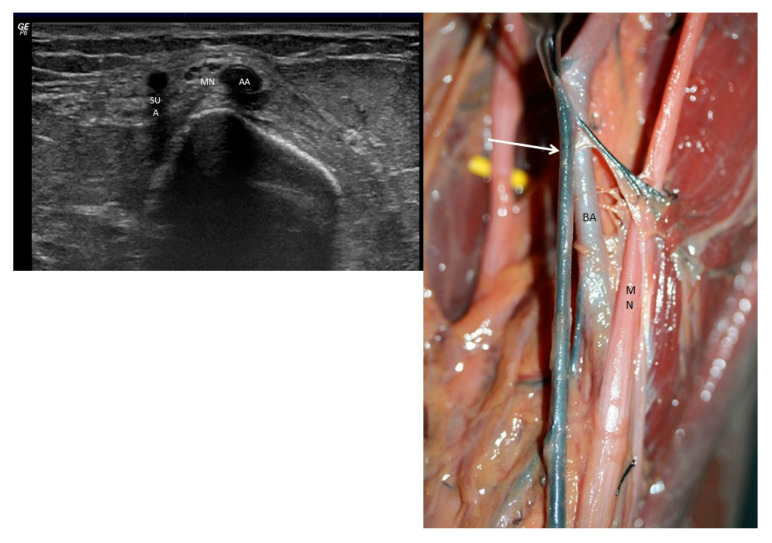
(Left) Ultrasound view in the elbow of the superficial ulnar artery (SUA) in a medial position from the median nerve (MN) and axillary artery (AA). (Right) Anatomic view of the median nerve (MN) that goes between the superficial ulnar nerve (white arrow) and brachial artery (BA).
